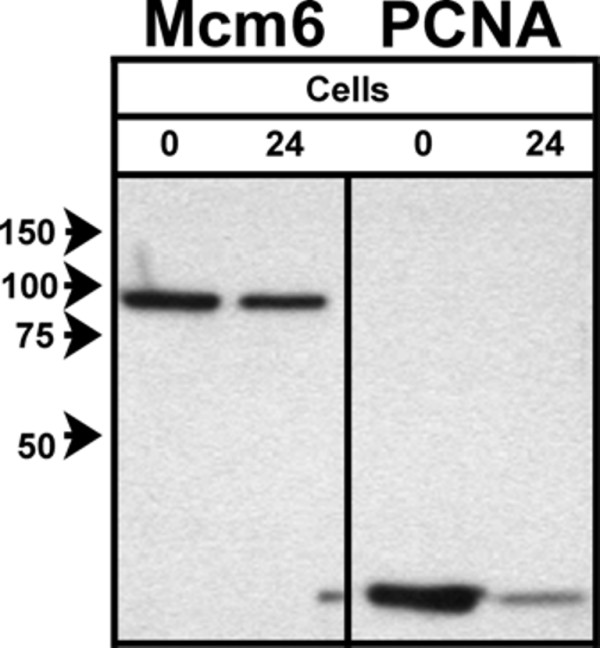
Cells in the low Mcm6 cluster express loaded Mcm6. BJ1 cells were harvested at 0 and 24 hours after serum starvation and prepared for electrophoresis after extracting the cells with Triton X-100 and washing. Equal cell numbers were loaded. The immunoblots were developed on X-Ray film that were then imaged and quantified. At 24 h, Mcm6 intensity was reduced by 25% and PCNA intensity by 72%. Under the conditions for serum starving BJ1 cells, there was an average of 5% of cells in S, G2 and M.
